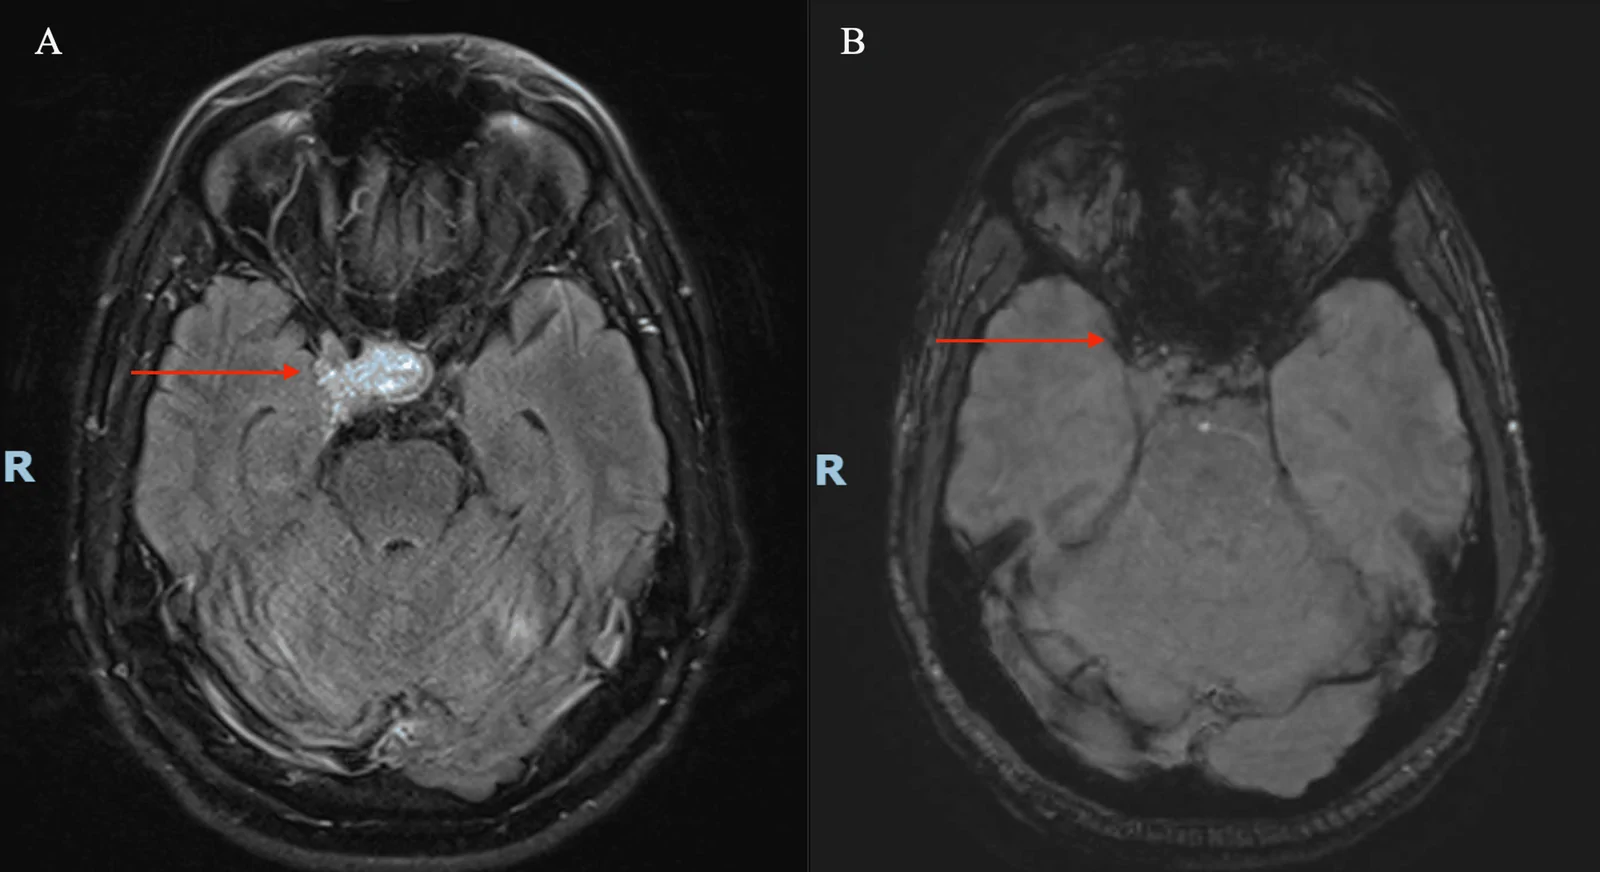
Pituitary macroadenoma visualised with fluid-level seen on T2 weighted fluid-attenuated inversion recovery (FLAIR) axial cuts (A), and blooming effect in the sellar region on susceptibility-weighted imaging (SWI) sequence (B), suggestive of pituitary apoplexy.
